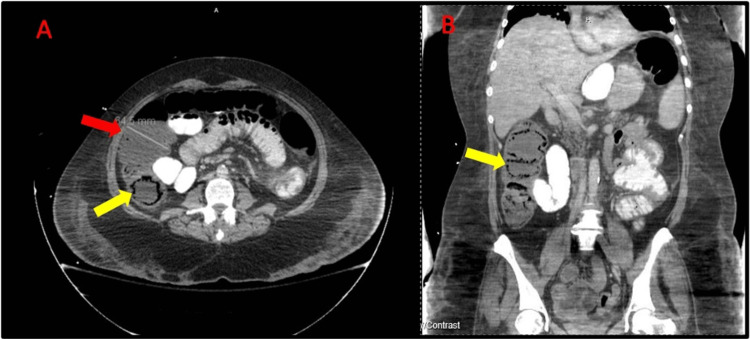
A: Initial CT (axial view) of the abdomen demonstrating pneumatosis coli (yellow arrow) and colonic distension (red arrow). B: CT scan (coronal view) showing pneumatosis coli of the ascending colon (yellow arrow). CT: computed tomography
